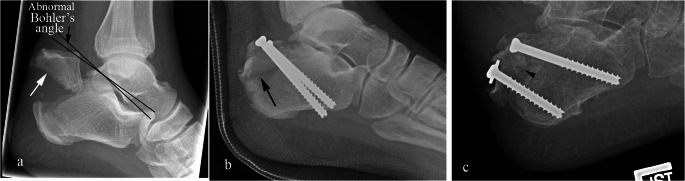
Type II calcaneal tuberosity avulsion fracture. Lateral radiographs of a 47-year-old man with calcaneal tuberosity avulsion fracture (white arrow) with an abnormal Bohler’s angle (black arrow) at presentation (a), clinically with skin tenting and blanching (not shown). Follow up radiograph (b) after percutaneous screw fixation resulted in persistent displacement of the fracture (black arrow in b). Follow up 11 months later (c) after revision with placement of new screws showing improved alignment of the osseous fragments (arrowhead)
